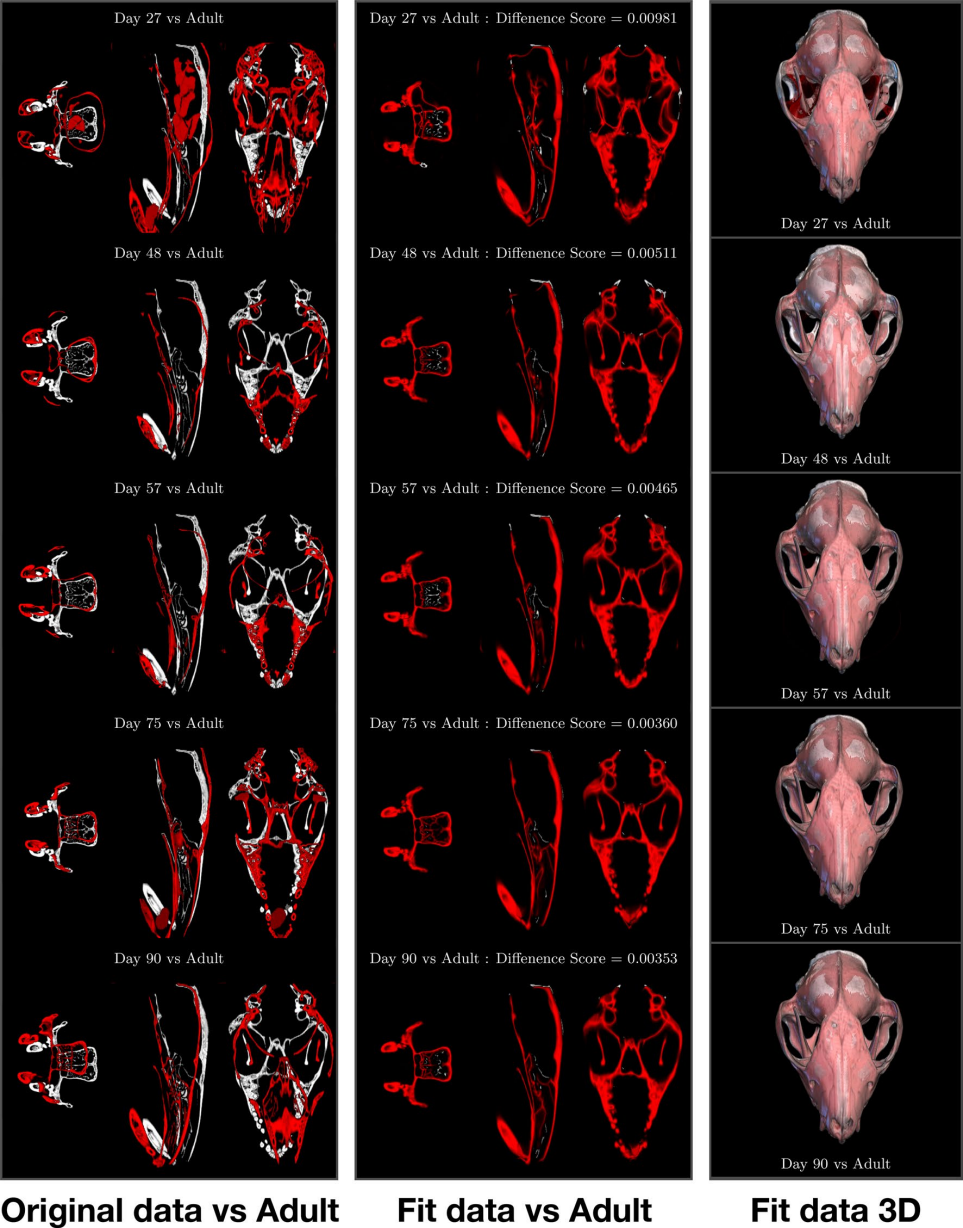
Volumetric SAPID analysis of high resolution X-ray CT images of Monodelphis. (Left) Slice through original volumetric CT data (red) overlayed with adult CT (grayscale), (Middle) Slice through volumetric non-linear registration of original CT data (red) overlayed with adult CT (grayscale), (Right) 3D visualization of volumetric non-linear registration of original CT data (red) overlayed with adult CT (grayscale).
